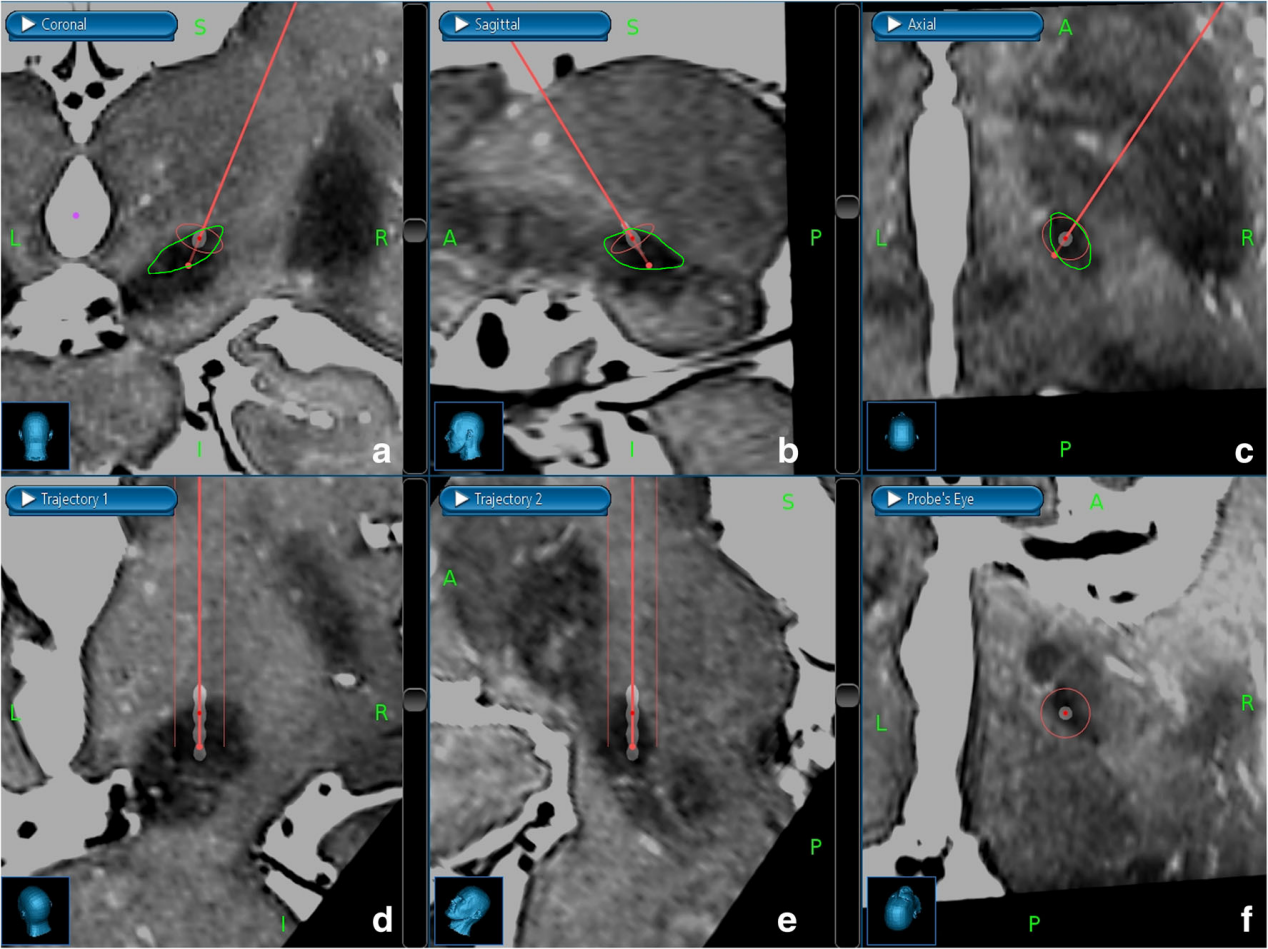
Direct targeting to the dorsolateral border of the subthalamic nucleus (STN, green line) in the right hemisphere. The preoperative stereotactic 3T-MRI T2-sequences and postoperative stereotactic CT scans are fused, and the placement of permanent lead is compared with that of the targeting plan and the location of STN. Upper row: The image fusion is shown in three radiological planes: coronal (a), sagittal (b), and axial (c). The targeting trajectory of the permanent DBS lead (thin red line) is shown with two red dots: the darker red dot (distal) is in the location where the second distal contact of the permanent lead is placed. The lighter red dot (proximal) is in the target point, which is between the most and second proximal contact. Lower row: The image fusion is shown in three planes (subfigures d, e, and f), and the viewing direction is parallel to the targeting trajectory and the permanent lead. All four contacts of the permanent lead are shown. The two middle contacts of the permanent lead are placed into the dorsolateral border of STN. The distal red dot is in the dorsal border, and the proximal red dot is in the ventral border of STN
